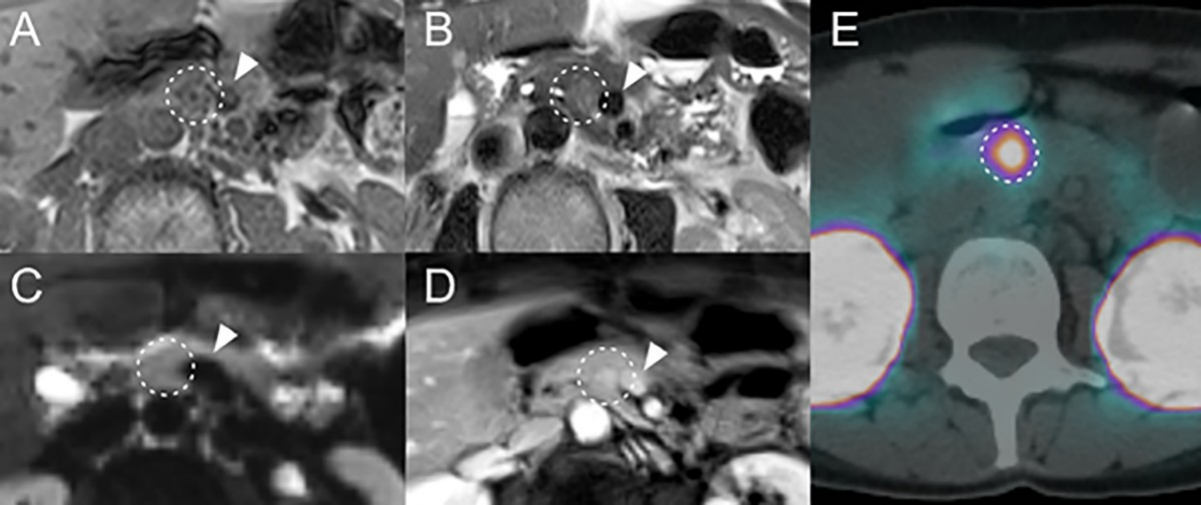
Imaging example of a small PICT with atypical signal characteristics. PICT localized in the pancreatic body (white dashed circle): isointense on in-phase T1w (A), slightly hyperintense to isointense on T2w HASTE (B), isointense on DWI b-value 800 s/mm^2^ (C) and faintly hyperenhancing to isoenhancing on ceGRASP (D). ^68^Ga-DOTA-exendin-4 PET/CT (E) confirming the lesion. The white arrowhead marks the superior mesenteric vein as reference.
